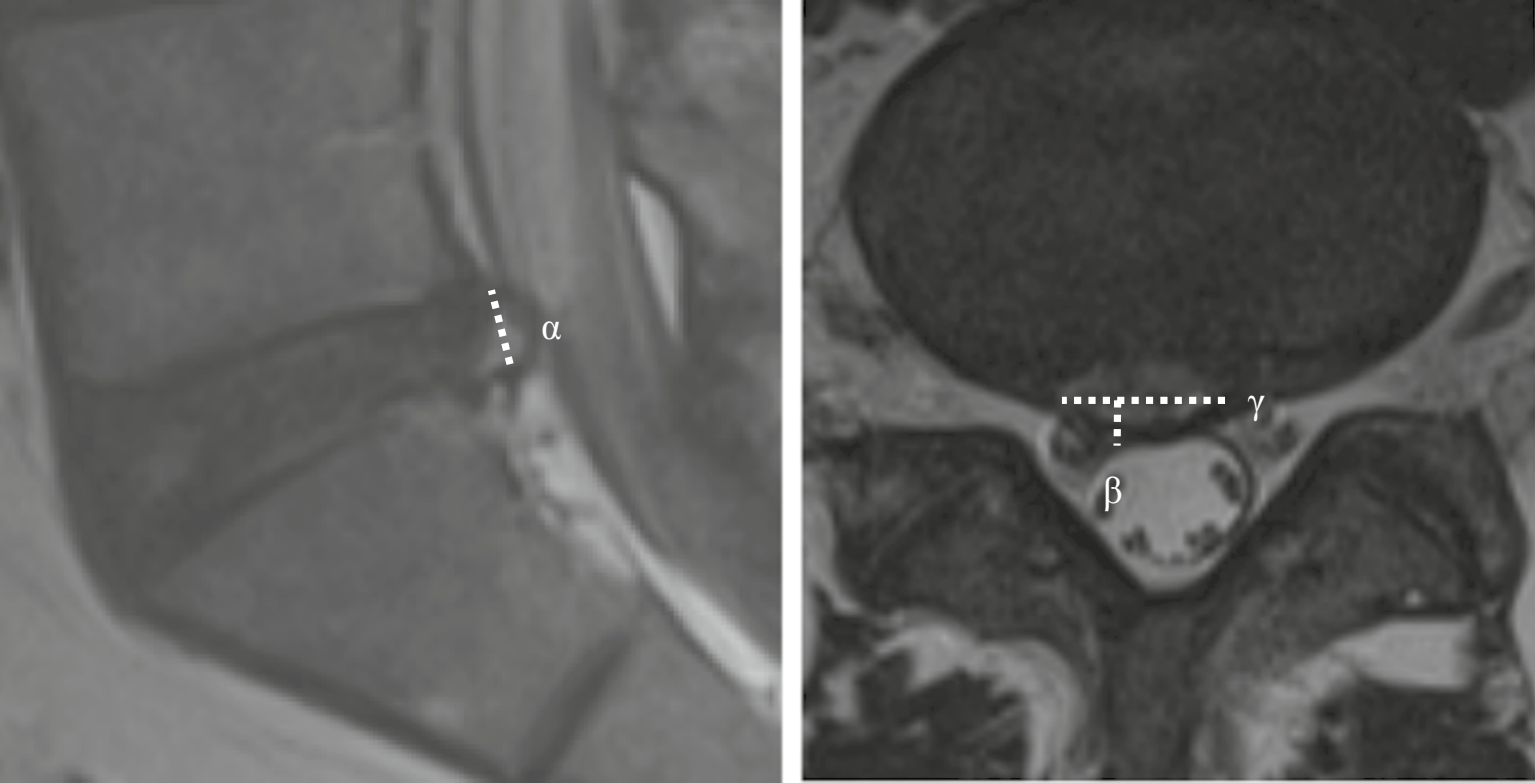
The protruded disc volume was determined as height (α) × depth (β) × transverse diameter (γ) of disc herniation × 1/2 (mm^3^) in preoperative magnetic resonance imaging
